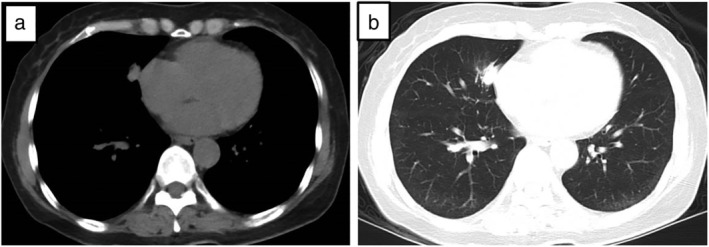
Chest CT scan demonstrated a right middle lobe space‐occupying lesion. a) Mediastinal window. (b) Lung window.
